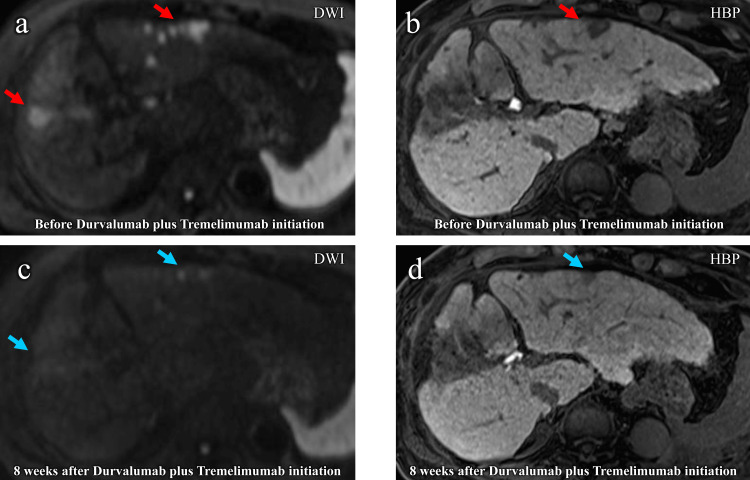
Image changes of MRI images before and after durvalumab plus tremelimumab initiation in Case 1 (a, b) are the diffusion-weighted image (DWI) and hepatobiliary phase (HBP) image before durvalumab plus tremelimumab initiation (red arrows indicate viable tumor); (c, d) are DWI and HBP images eight weeks after durvalumab plus tremelimumab initiation (blue arrows indicate shrunken viable tumor).
